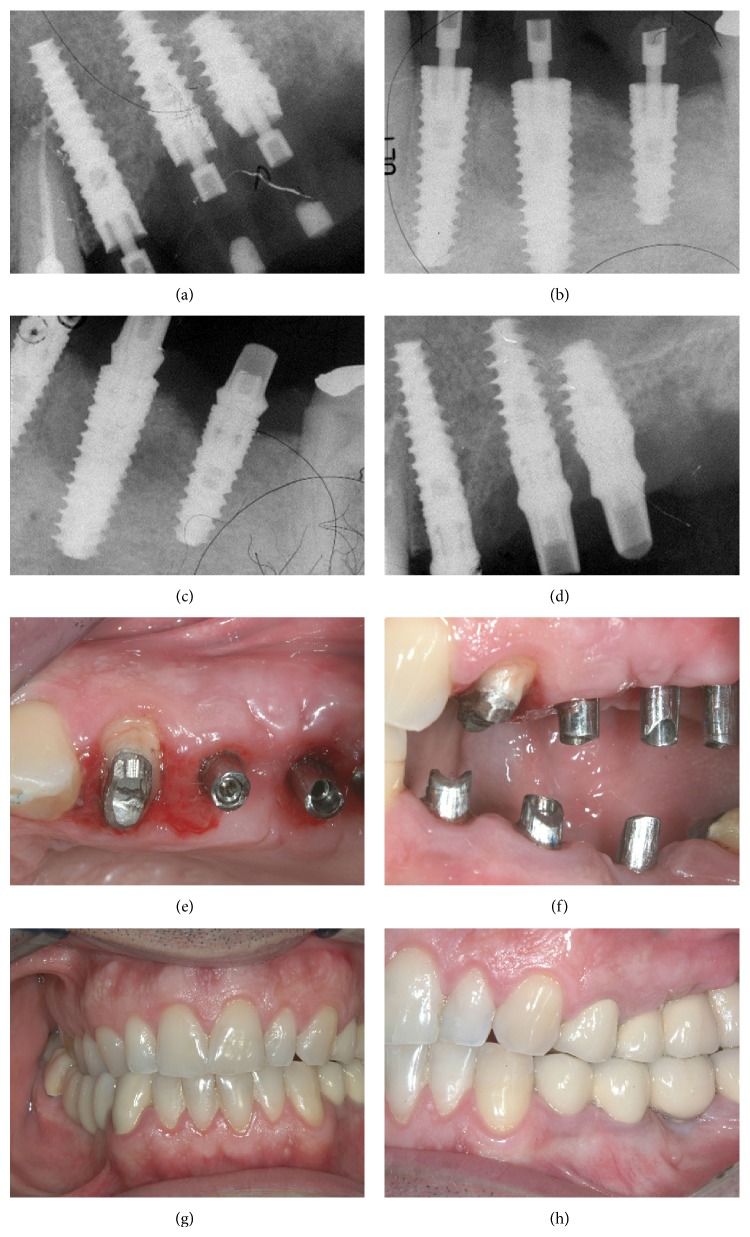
X-rays showing good implants/abutments contact and final prosthesis.
